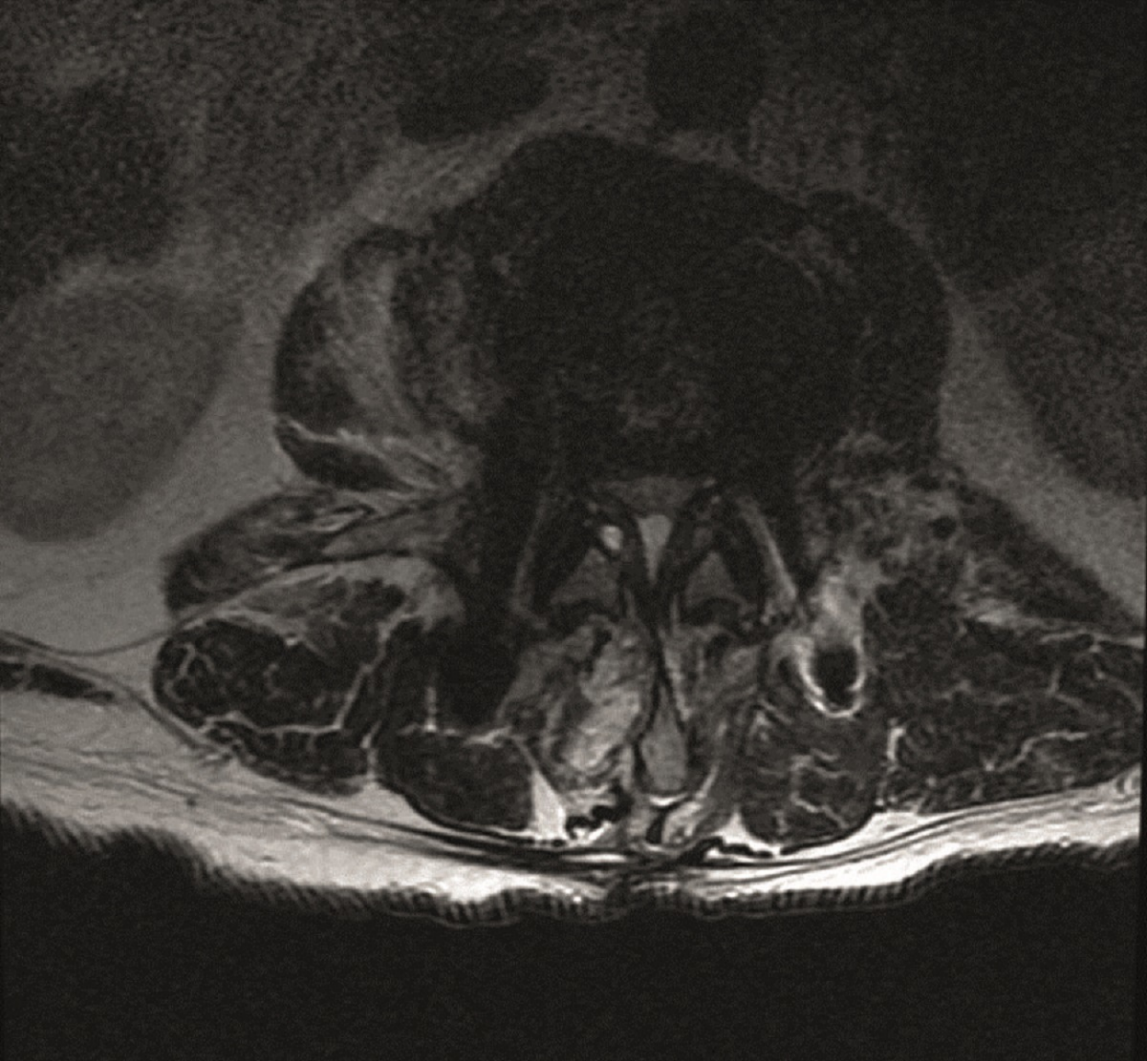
Axial T2 Pre-operative MRI Severe spinal canal stenosis at L2-L3 in association with a disc bulge and ligamentum flavum thickening and a small cyst on the right side.
